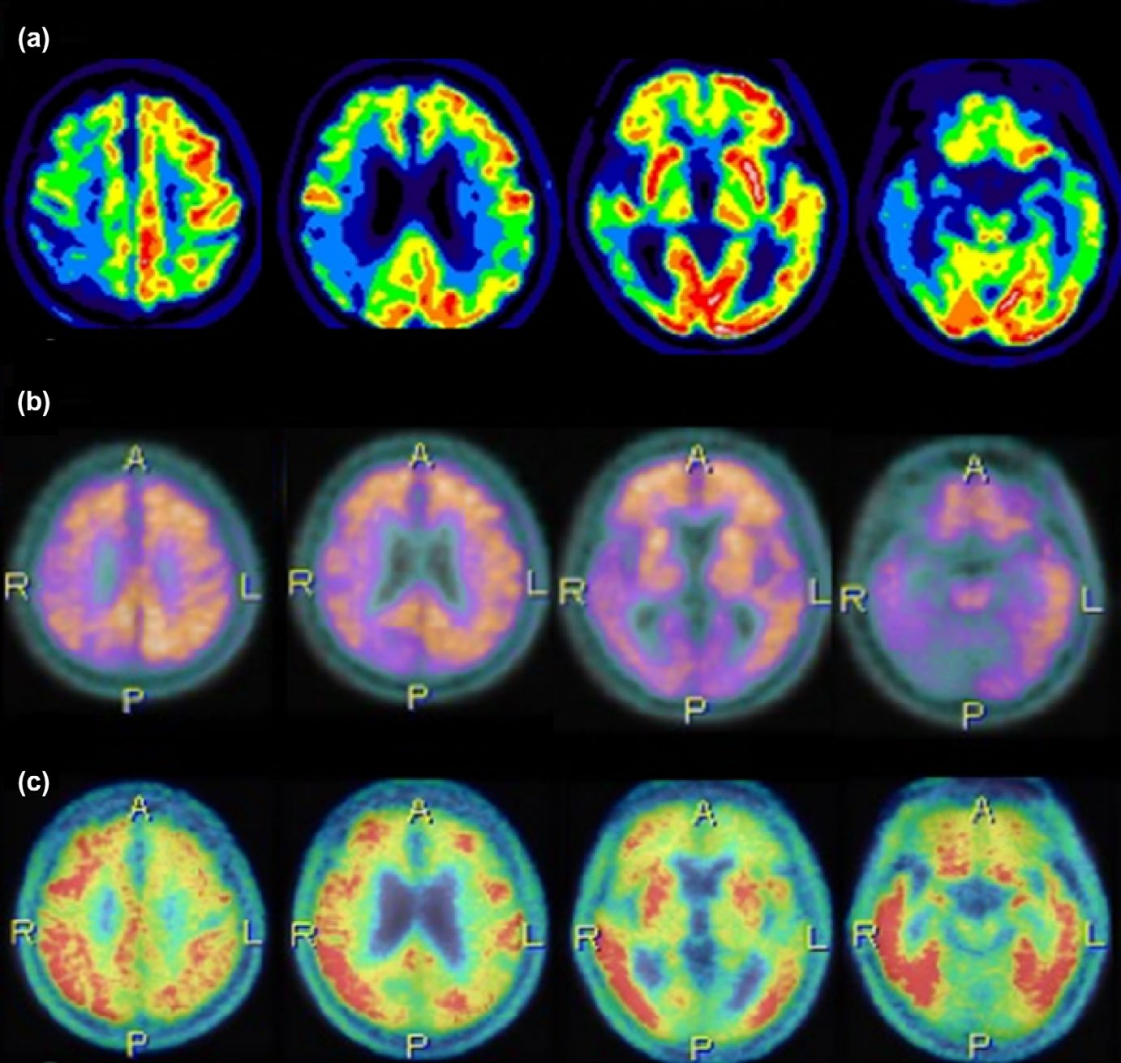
Brain FDG‐PET, ^11^C‐PiB amyloid PET, and ^18^F‐T807 tau PET image findings of the index patient. (a) FDG‐PET shows significant hypometabolism in the right frontal, parietal, occipital, and temporal regions and left lower parietal and superior temporal regions. (b) ^11^C‐PiB amyloid PET shows diffuse tracer retention in bilateral cerebral cortices, relatively lower in the right hemisphere and more obvious in frontal, parietal, precuneus, and anterior and posterior cingulate regions. (c) ^18^F‐T807 tau PET shows significant tracer retention in right frontal, parietal, and temporal regions and milder retention in the left parietal regions. The asymmetric topography was correlated with the hypometabolism pattern on FDG‐PET (a). FDG, metabolic fluorodeoxyglucose; PET, positron emission tomography; ^11^C‐PiB, ^11^C‐labeled Pittsburgh compound B
